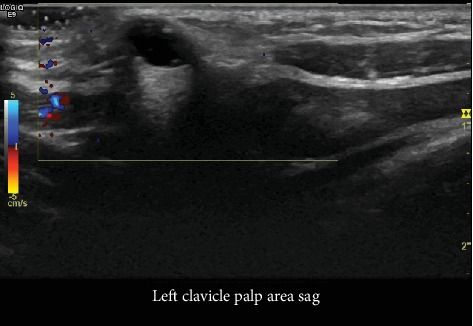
Single sonographic image with color Doppler of the left sternoclavicular joint demonstrates a circumscribed, avascular, cystic mass with a narrow neck that dives into the sternoclavicular joint. This connection with the joint is typical for ganglia.
